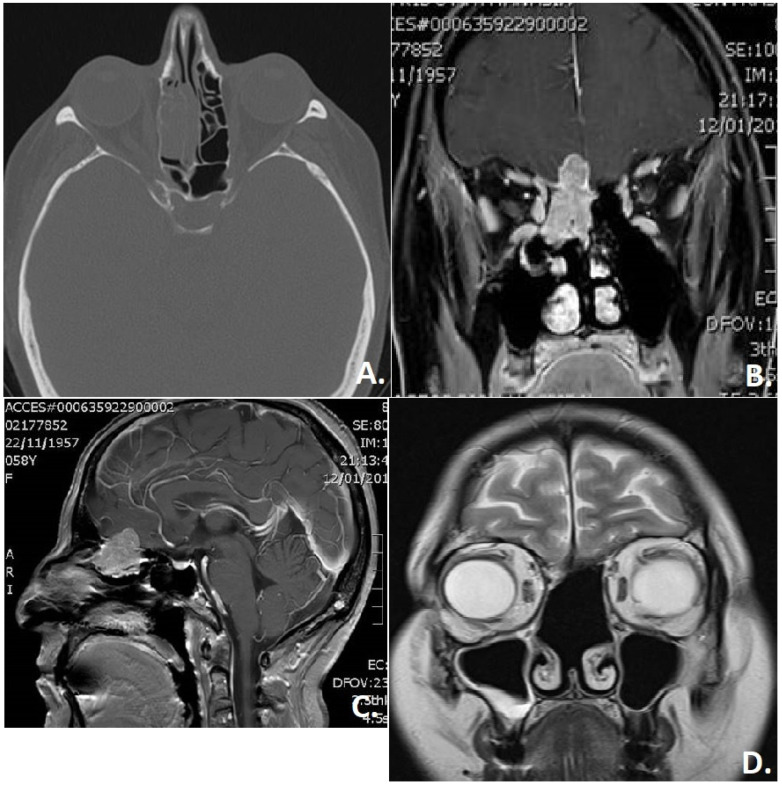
Imaging of a patient with an olfactory neuroblastoma. (A) Computed tomography (axial section) shows involvement of the ethmoid cells on the right side. (B) Magnetic resonance imaging (coronal section) and (C) sagittal section shows a marked “nodular” intracranial extension of the tumor. (D) Follow-up: magnetic resonance imaging (coronal section) without sign of local recurrence on follow-up.
